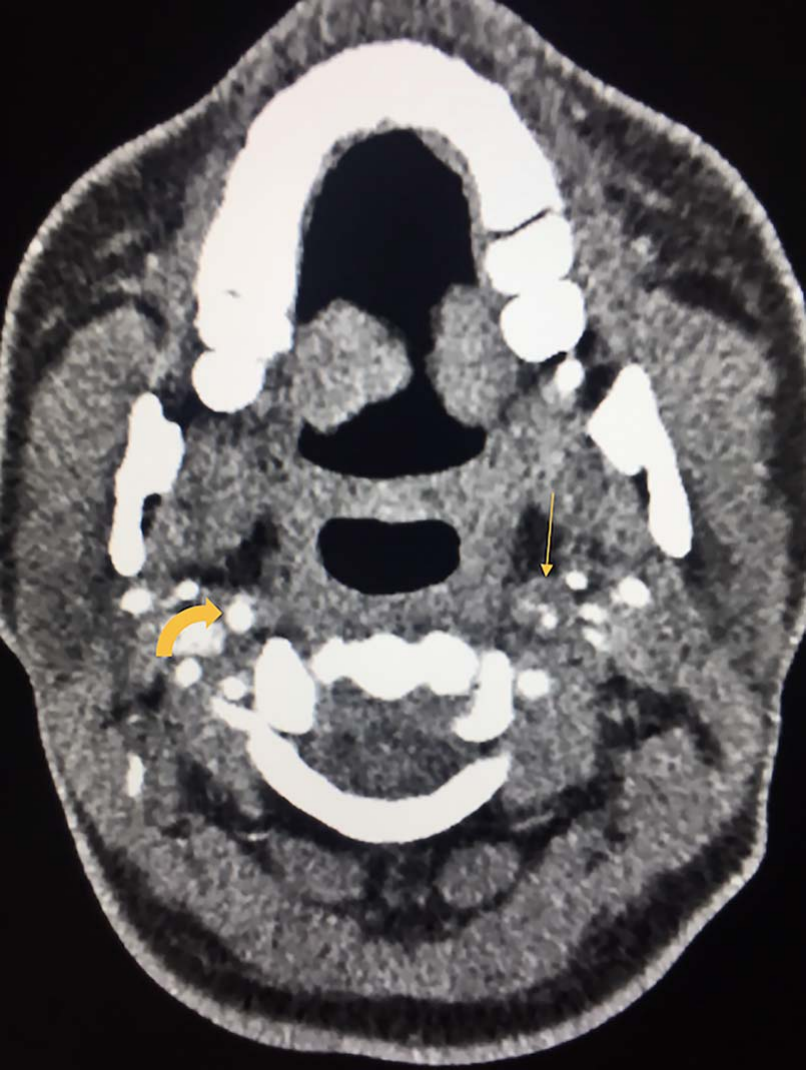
Axial image on CT angiogram shows enlargement of the left ICA diameter with a narrowed eccentric lumen compressed by the approximately isoattenuating intramural hematoma (straight arrow) relative to the surrounding muscle. This is in comparison to the normal enhancement of the right ICA (curved arrow).
